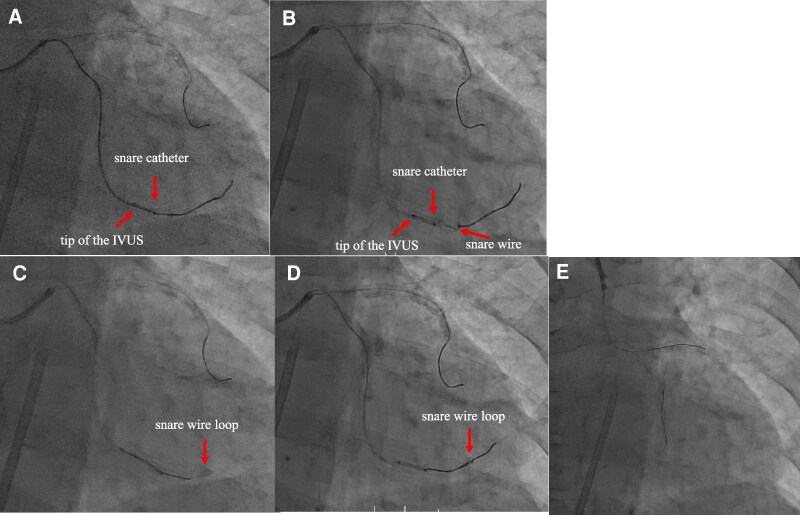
Retrieval of the remaining intravascular ultrasound distal imaging core shaft. (*A* and *B*) Delivery of the snare catheter to the target site using the modified method. (*C*) Manipulate the original guidewire loading into the distal imaging core shaft to pass the gooseneck snare wire loop. (*D*) The original guidewire goes through the loop. (*E*) After retrieval of the intravascular ultrasound distal imaging core shaft.
